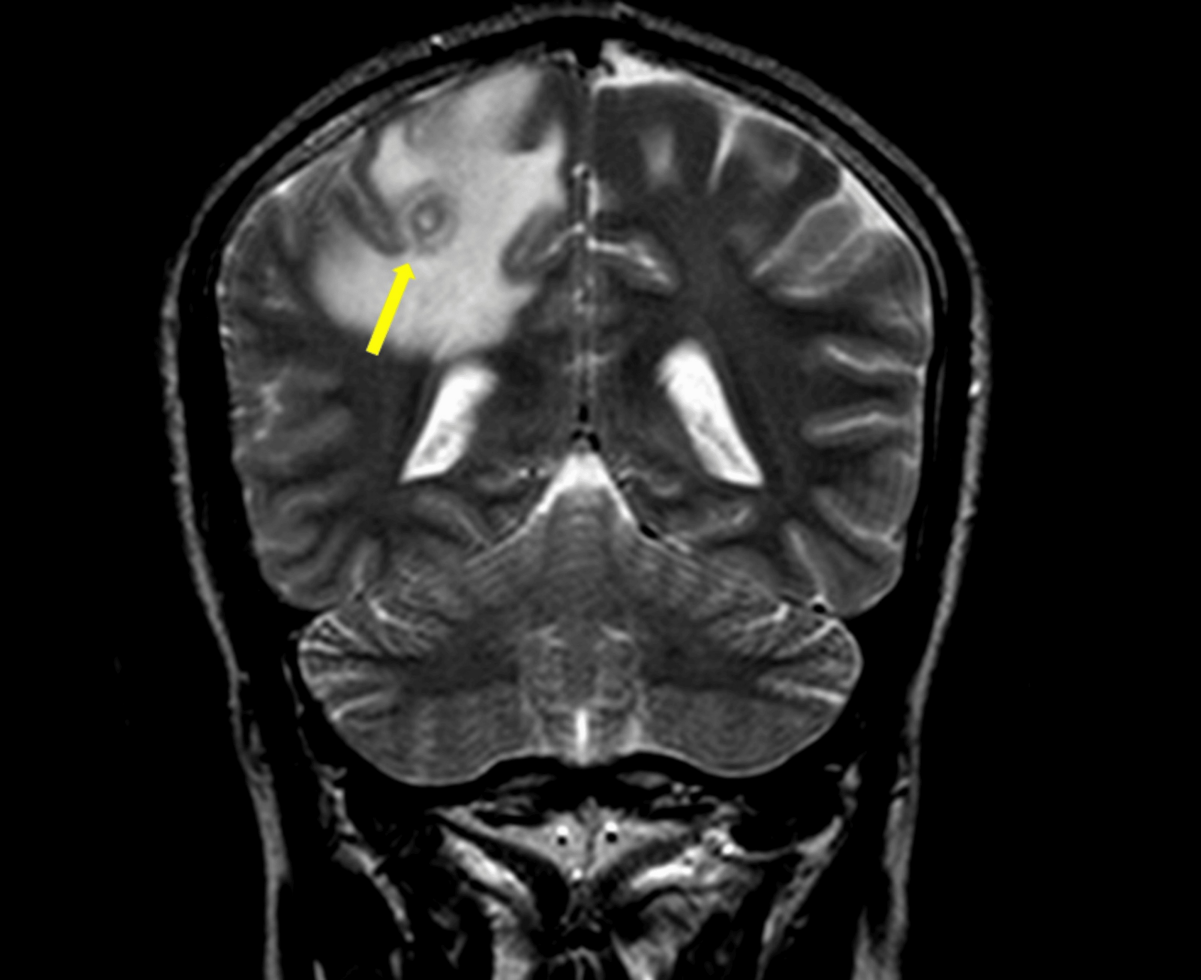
Coronal Brain MRI showing subcortical grapelike ring-enhancing lesions in the right parietal lobe with surrounding right fronto-parietal lobe vasogenic oedema. These features are consistent with the colloidal vesicular stage of NCC.
